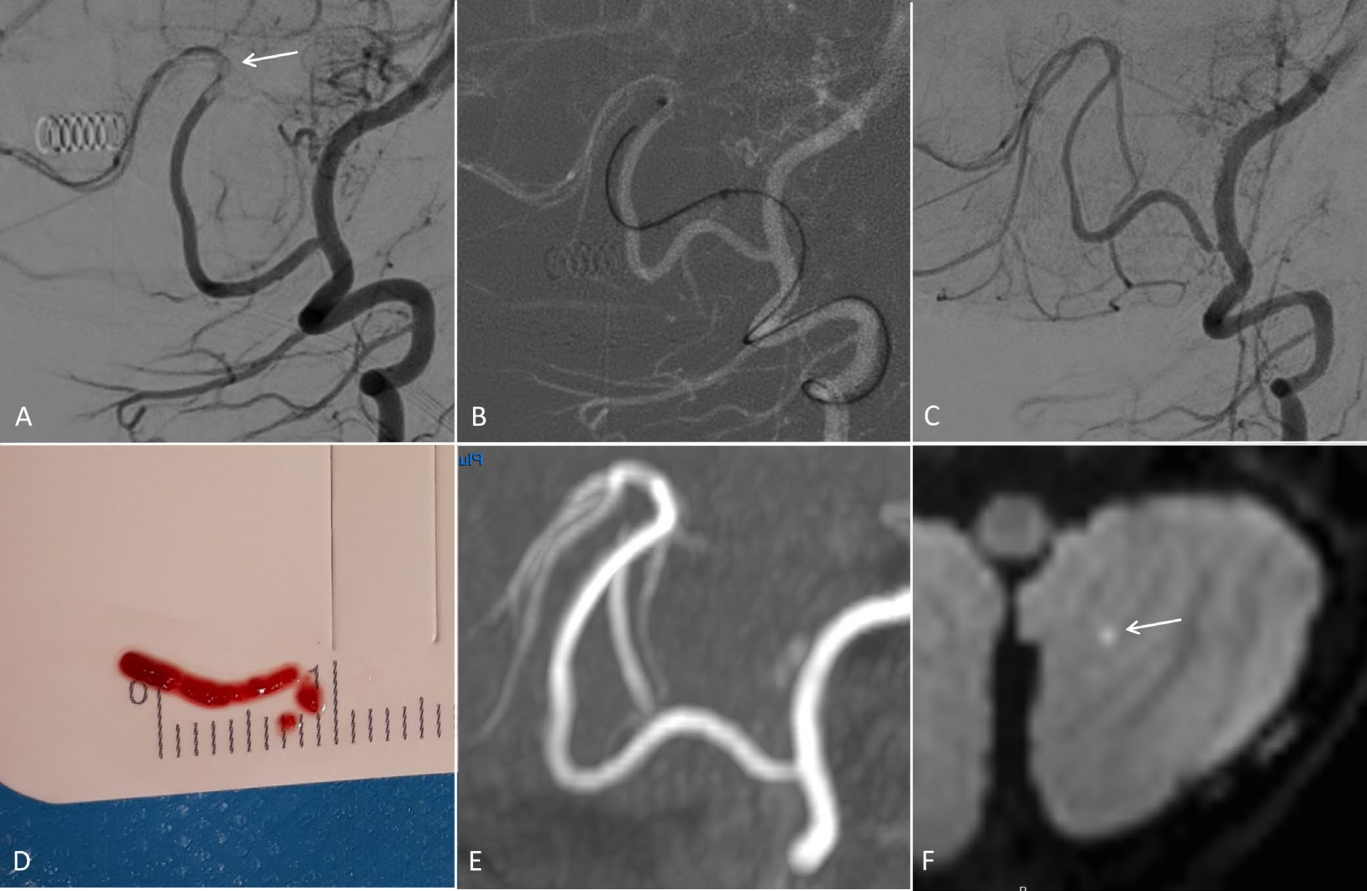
Patient 1, 11‐months‐old. Clot embolus in the PICA (A: Arrow) treated by direct thromboaspiration through a Phenom 0.027 microcatheter (B) with an eTICI score of 3 after 2 passes (C). The clot (D) was stuck to the tip of the microcatheter. MRA the day after (E) showed a patent PICA with only a small spot on DWI (F: Arrow). The pedNIHSS was 0 when the patient woke up after the intervention.
